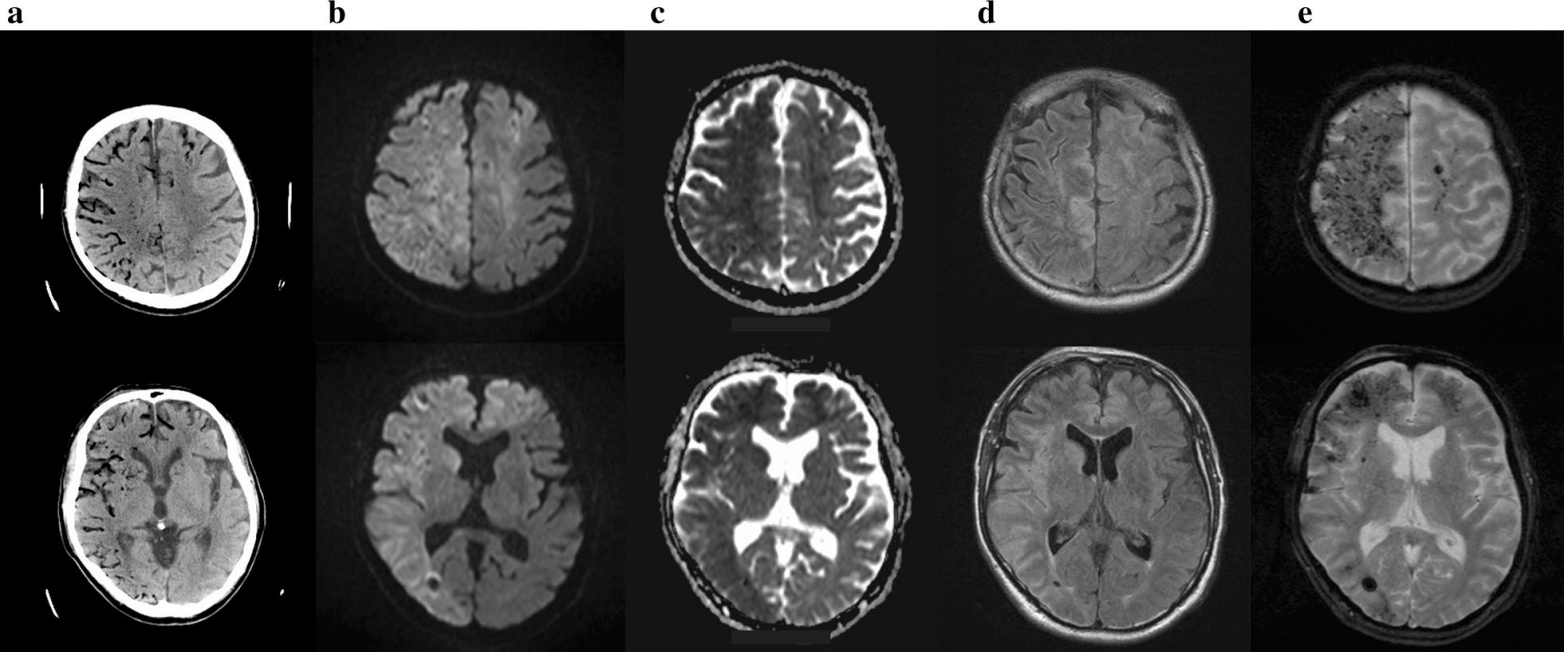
Brain CT and MRI of case 1. a Brain CT shows numerous foci of intravascular air within the area supplied by bilateral anterior cerebral arteries (ACAs) and the right middle cerebral artery (MCA). b Diffusion-weighted imaging (DWI) and d fluid attenuated inversion recovery (FLAIR) images show high-intensity areas that are supplied by the bilateral anterior cerebral arteries and the right middle cerebral artery. c Hypointense signal was observed on the apparent diffusion coefficient (ADC) map. e T2 star-weighted imaging (T2*WI) shows many hypointense spots in the area perfused by the bilateral anterior cerebral arteries and the right middle cerebral artery
